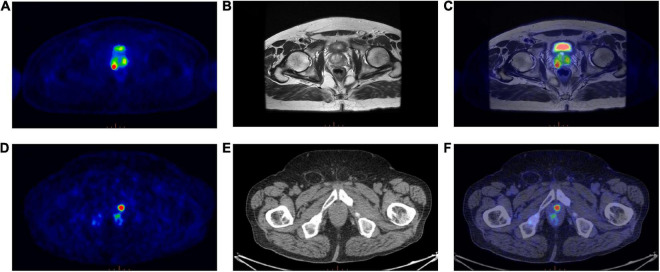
(Top panel) Exemplar image of a ^68^Ga-PSMA PET/MRI scan; (A) Transaxial ^68^Ga-PSMA PET, (B) Axial T2-weighted MRI sequence, (C)
